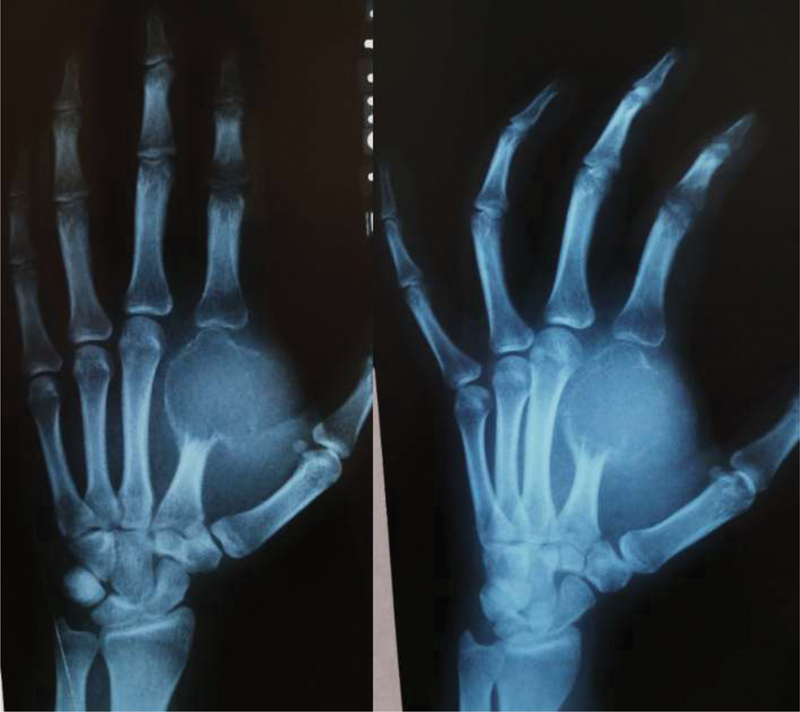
Case 1: frontal and oblique radiographs of the left hand two months after incisional biopsy by an oncologic surgeon. Notice that the exaggerated opening of the pseudocapsule enabled the rapid expansion of the tumor to the soft tissues.
